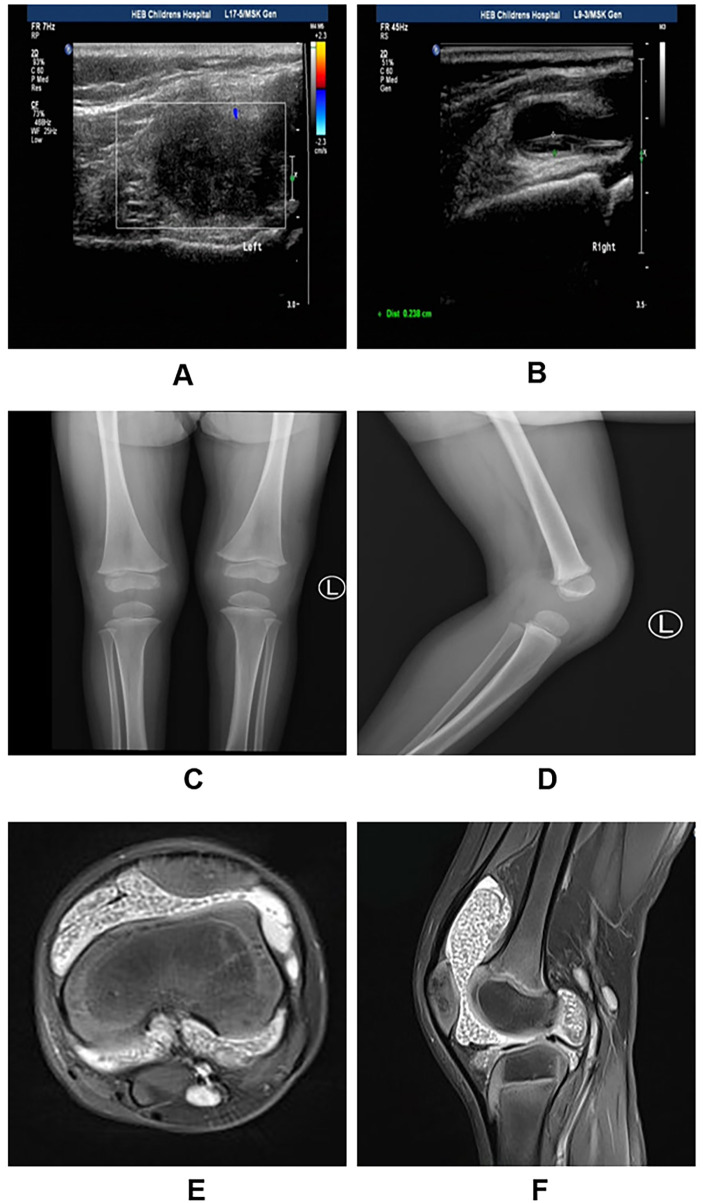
Multimodal preoperative imaging assessment of pediatric rice body synovitis. (A,B) Ultrasonography revealing marked synovial thickening with multiple hypoechoic nodules floating within the effusion. (C,D) Anteroposterior and lateral radiographs showing significant soft tissue swelling (distension of the suprapatellar pouch) without bone erosion. (E,F) Magnetic Resonance Imaging. (E) T2-weighted sagittal image demonstrates a massive joint effusion containing numerous hypointense, discrete nodules (white arrows). (F) T1-weighted axial image shows isointense nodules filling the joint capsule. Note the absence of blooming artifact, helping to distinguish from PVNS.
